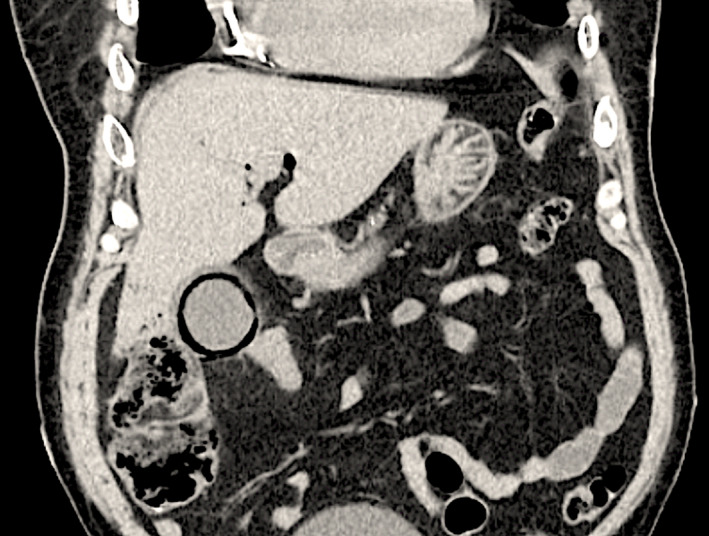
Coronal section view of CT abdomen showing thickening of the gall bladder wall surrounding emphysematous changes. Also seen are calcific pancreatitis and distended urinary bladder
